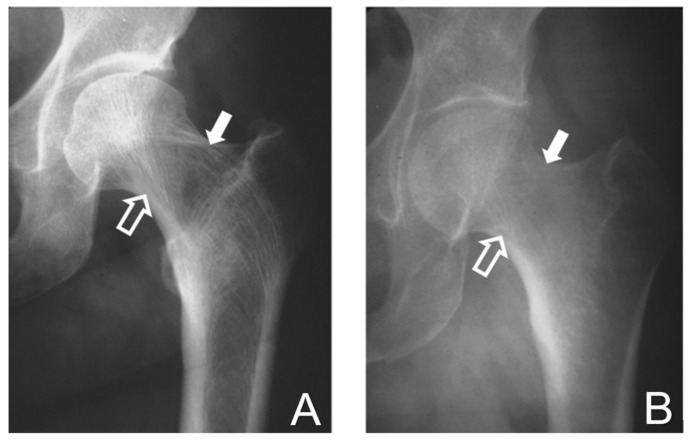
Anteroposterior radiographs of the human proximal femur showing the response to physiological loading (A) and osteoporosis (B). (A) In the normal femoral head, trabeculae align along lines of weight-bearing stress and tissue strain patterns. Compressive trabeculae (open arrows) transmit strain from the joint reactive forces to the medial femoral cortex. Tensile trabeculae (solid arrows) align to resist bending moments due to the offset of the center of rotation of the femoral head from the femoral shaft and transmit bending stress to the lateral femoral cortex. (B) In the osteoporotic femoral head, osteoblasts are not responsive to mechanical strain patterns and the trabecular orientation is diminished or lost. Adapted with permission from Ref. [3]. 2022, *Rhode Island Medical Journal*.
